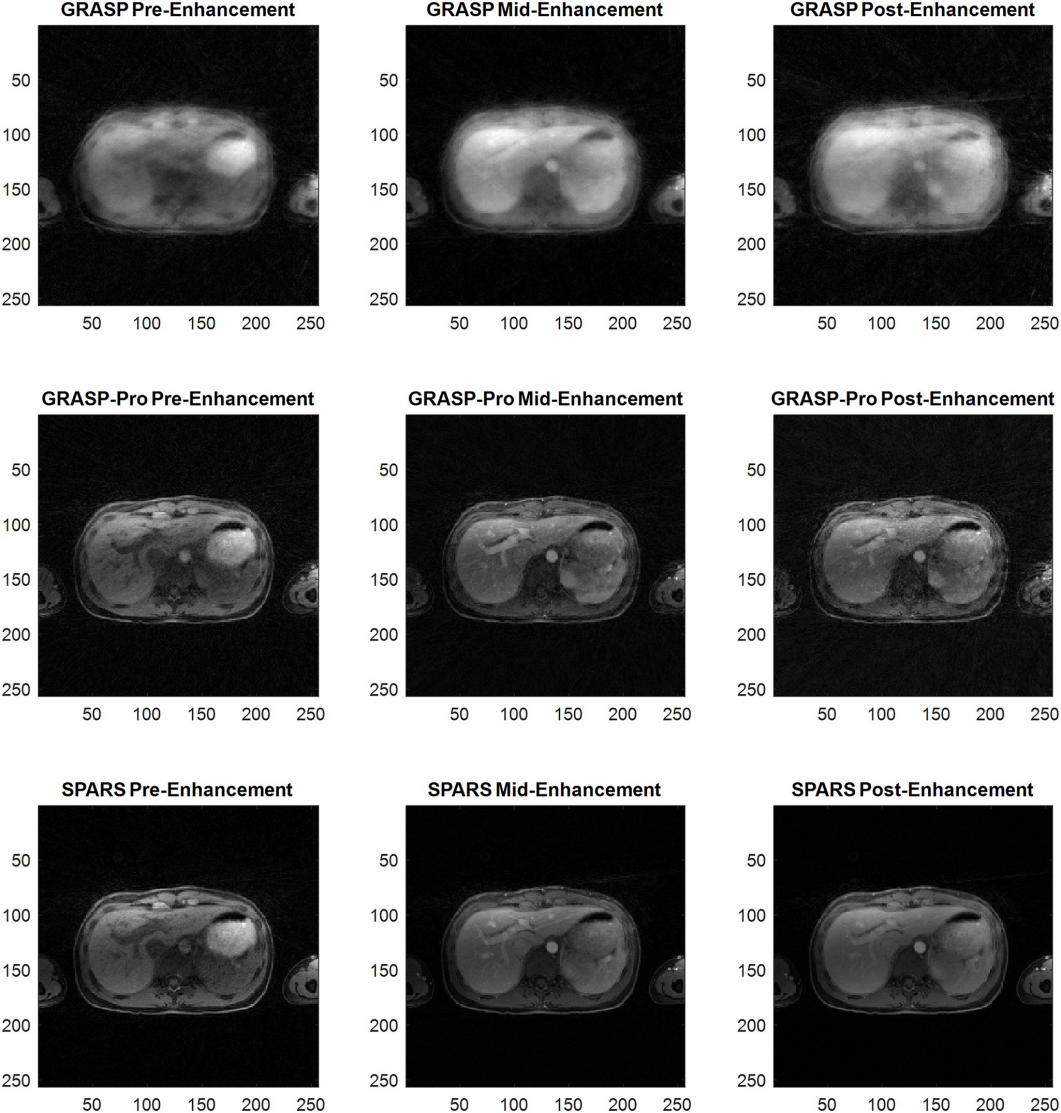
Reconstructed images of in-vivo human liver DCE-MRI for dataset 2. Comparison of GRASP, GRASP-Pro, and SPARS. Select timepoints are shown before, during, and after signal enhancement.
